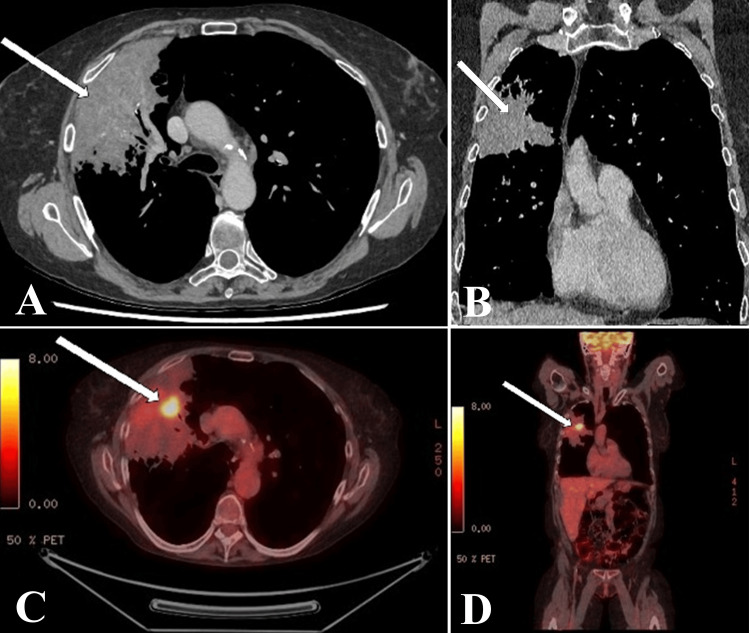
Diagnostic imaging (A) Axial and (B) coronal views of the initial diagnostic chest computed tomography scan. (C) Axial and (D) coronal views of the staging positron emission tomography-computed tomography scan. The white arrows indicate the hypermetabolic primary tumor with surrounding non-avid atelectasis.
